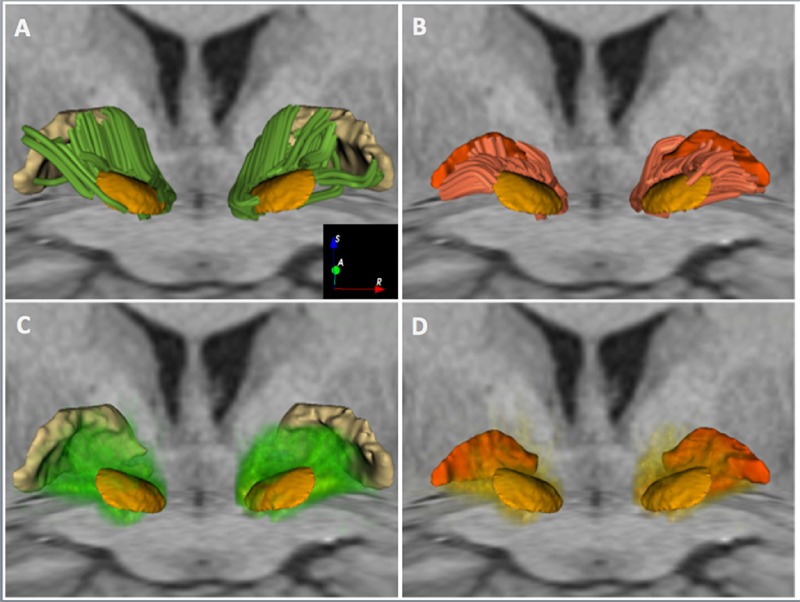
White matter connectivity between the STN and the GP in HCP subject 100307. The figure shows an anterior 3D view of MC-det tracts represented as streamlines (top row) and CSD-prob tracts represented as volume-rendered tract density maps (bottom row). The tracts are superimposed on an axial T1-weighted image at the level of the cerebral peduncles and a coronal T1-weighted image at the level of the anterior commissure, along with 3D surface models of the STN (light orange), GPi (dark orange), and GPe (light yellow). (A) and (C) show tracts interconnecting the STN and the GPe. (B) and (D) show tracts interconnecting the STN and the GPi.
